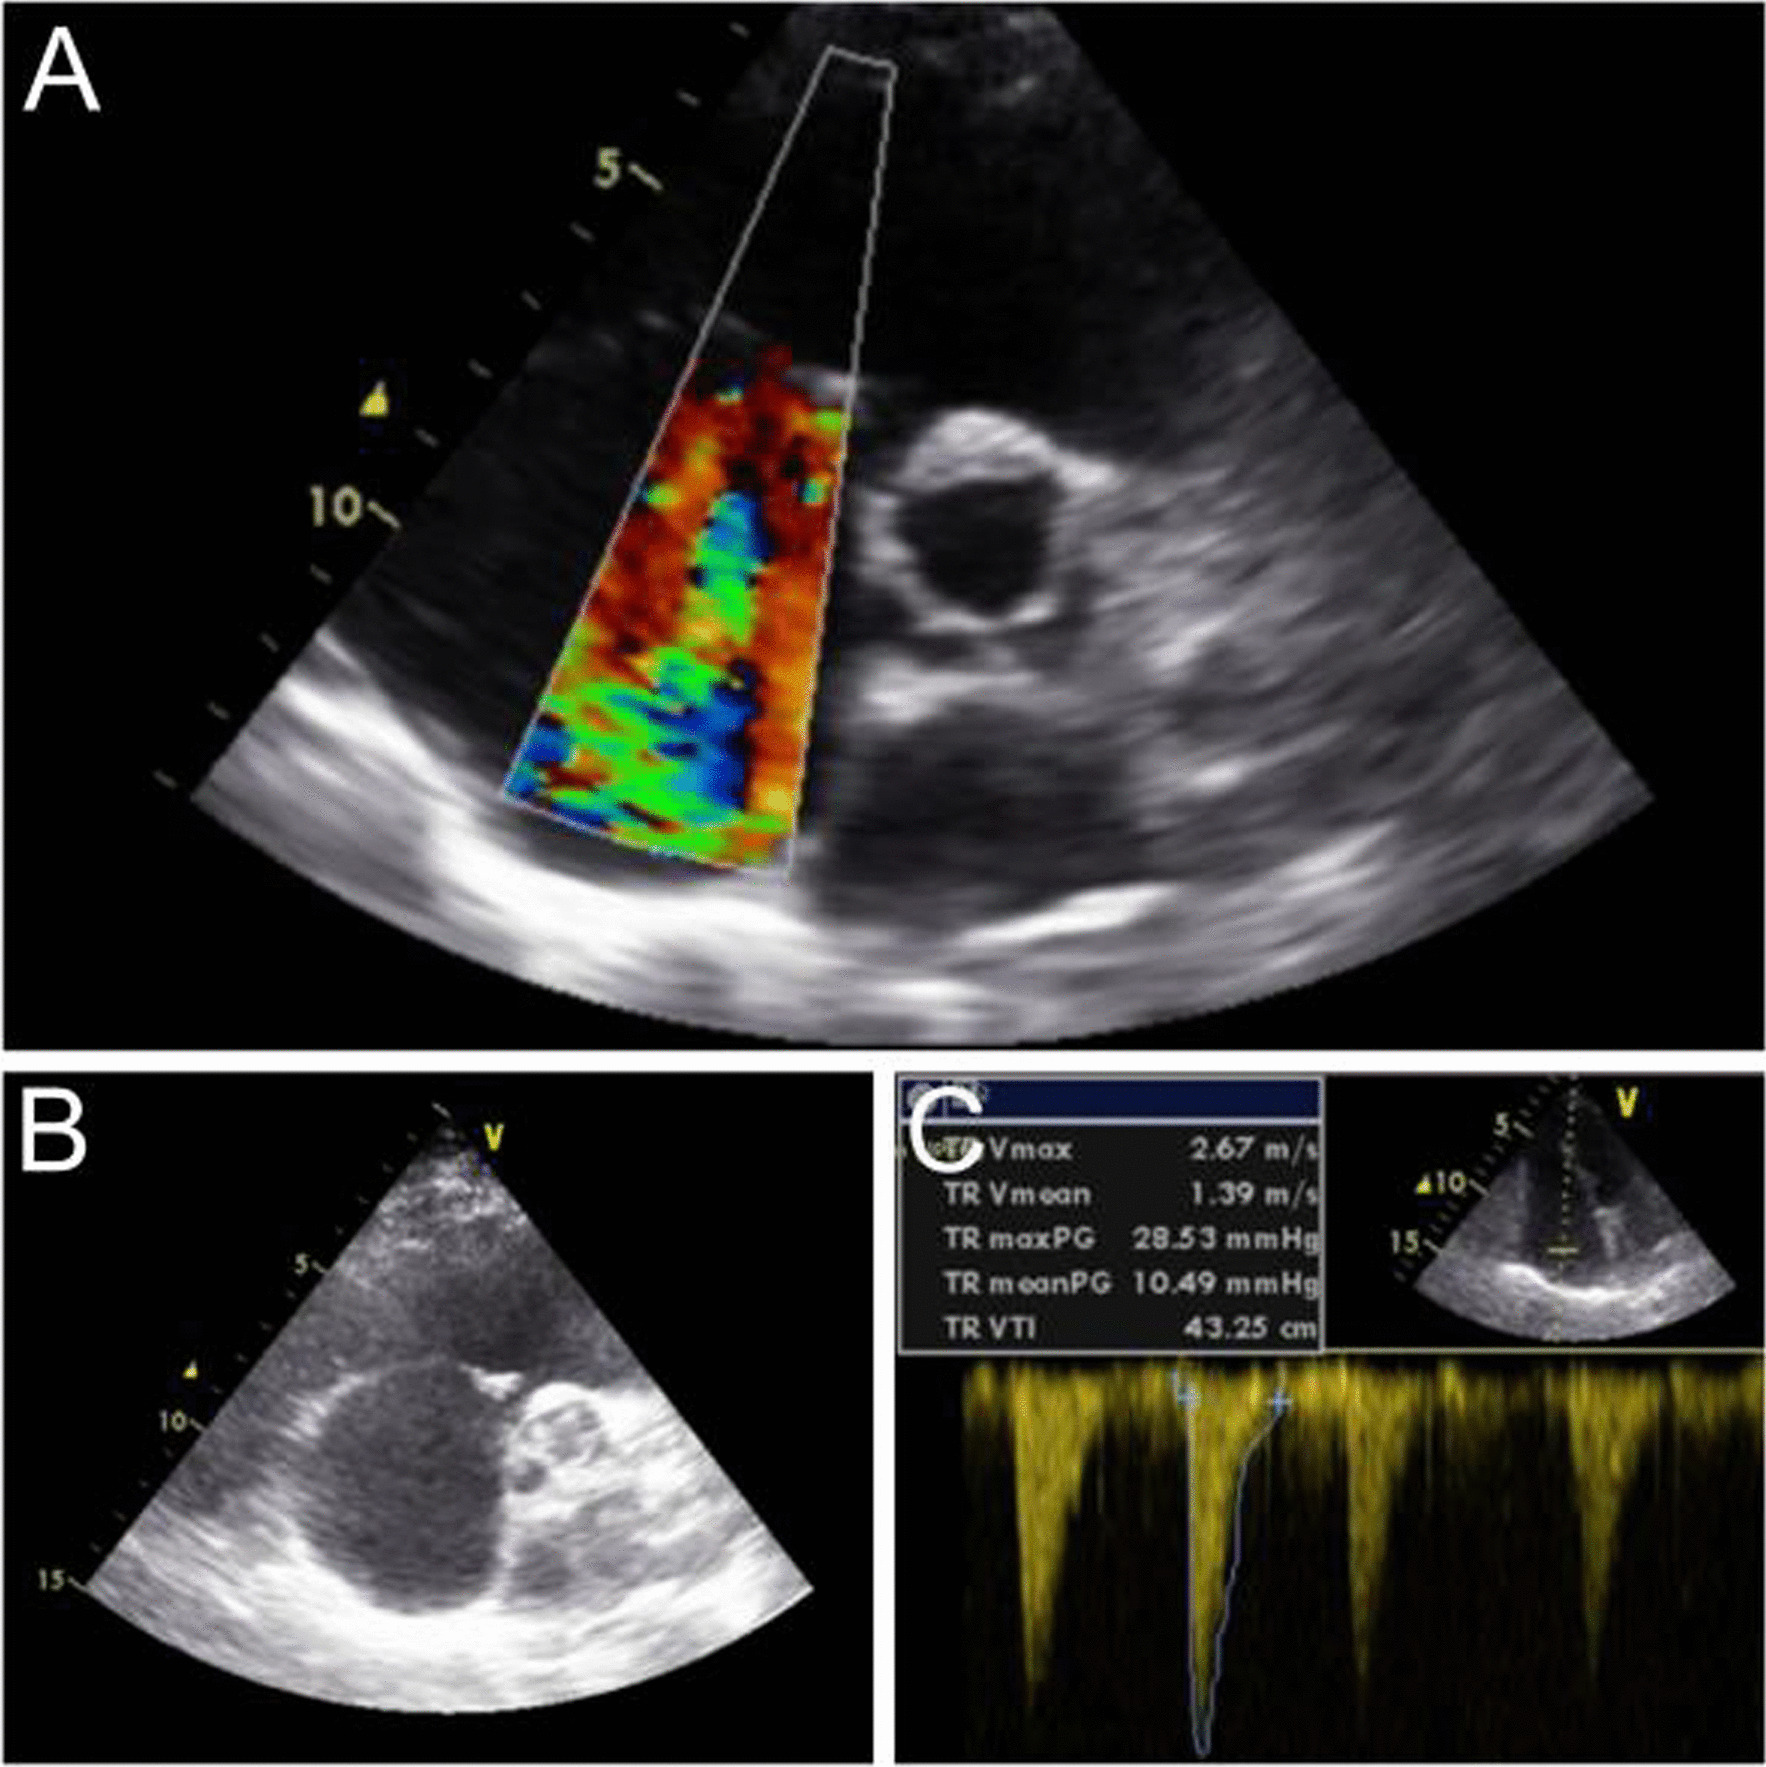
A Transthoracic echocardiogram (TTE) in parasternal short axis view with evidence of severe tricuspid regurgitation. B TTE in parasternal short axis view shows a dilated right heart. C Continuous wave spectral Doppler analysis of the tricuspid regurgitation (TR) jet for assessing pulmonary arterial pressure
